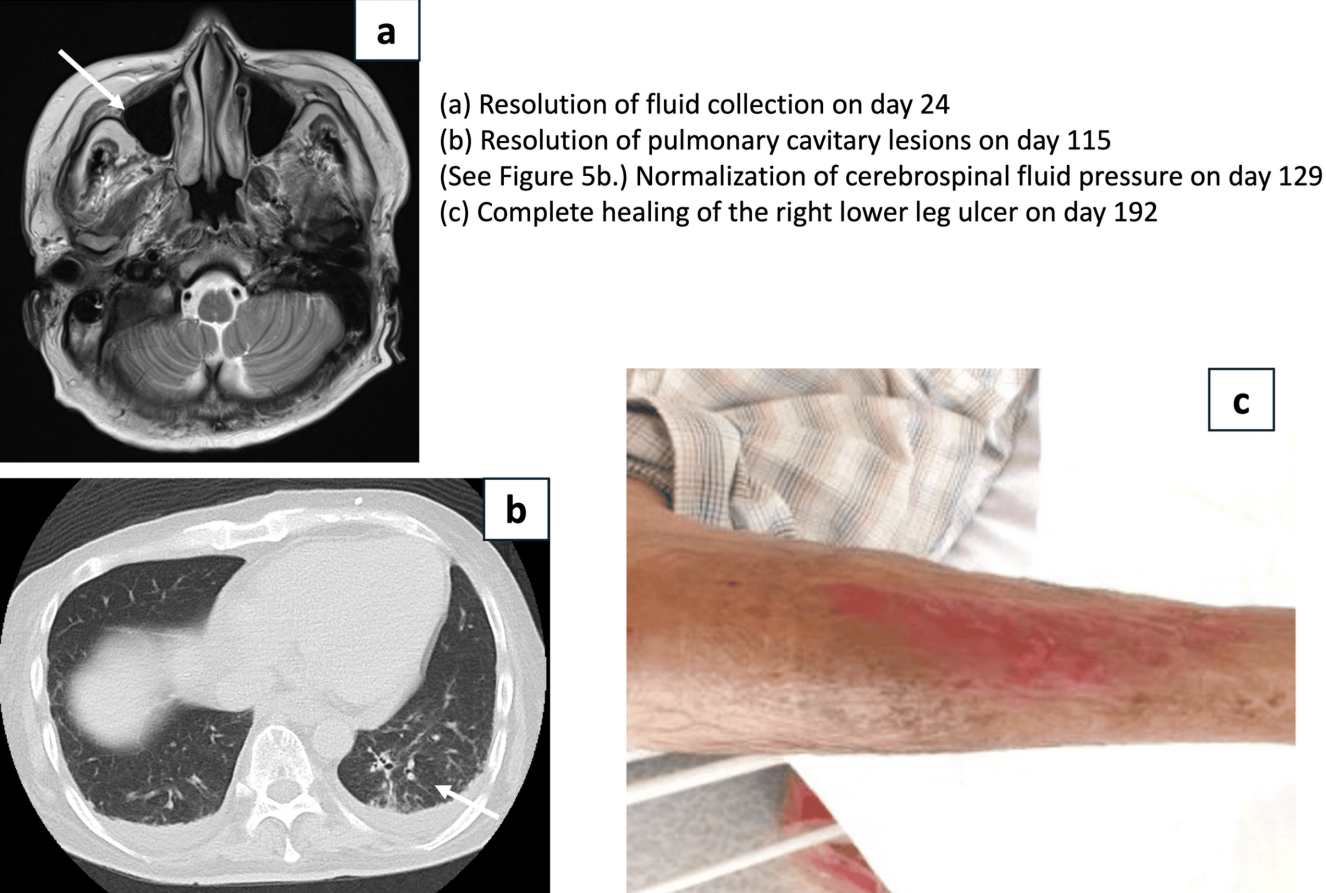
Time to improvement of each symptom (a) On the head MRI obtained on day 24, improvement of the fluid collection indicated by the white arrow was confirmed. (b) On the chest CT obtained on day 115, improvement of the cavitary nodular lesion in the left middle lobe, indicated by the white arrow, was confirmed. (c) On day 192, complete epithelialization of the entire ulcer of the right lower leg was observed. On day 129 after ventriculoperitoneal (VP) shunt placement, the cerebrospinal fluid pressure had normalized, and no further lumbar punctures were performed after day 135 (see Figure 5b).
